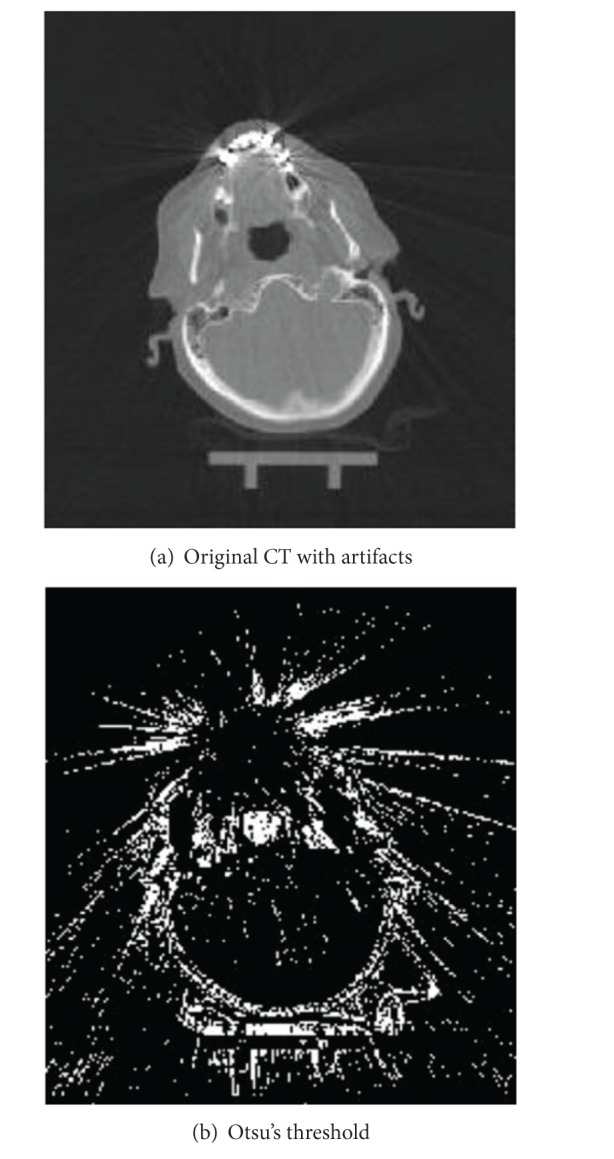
Original CT image with dental streaking artifacts (a) and detected artifacts with Otsu's thresholding method (b).
